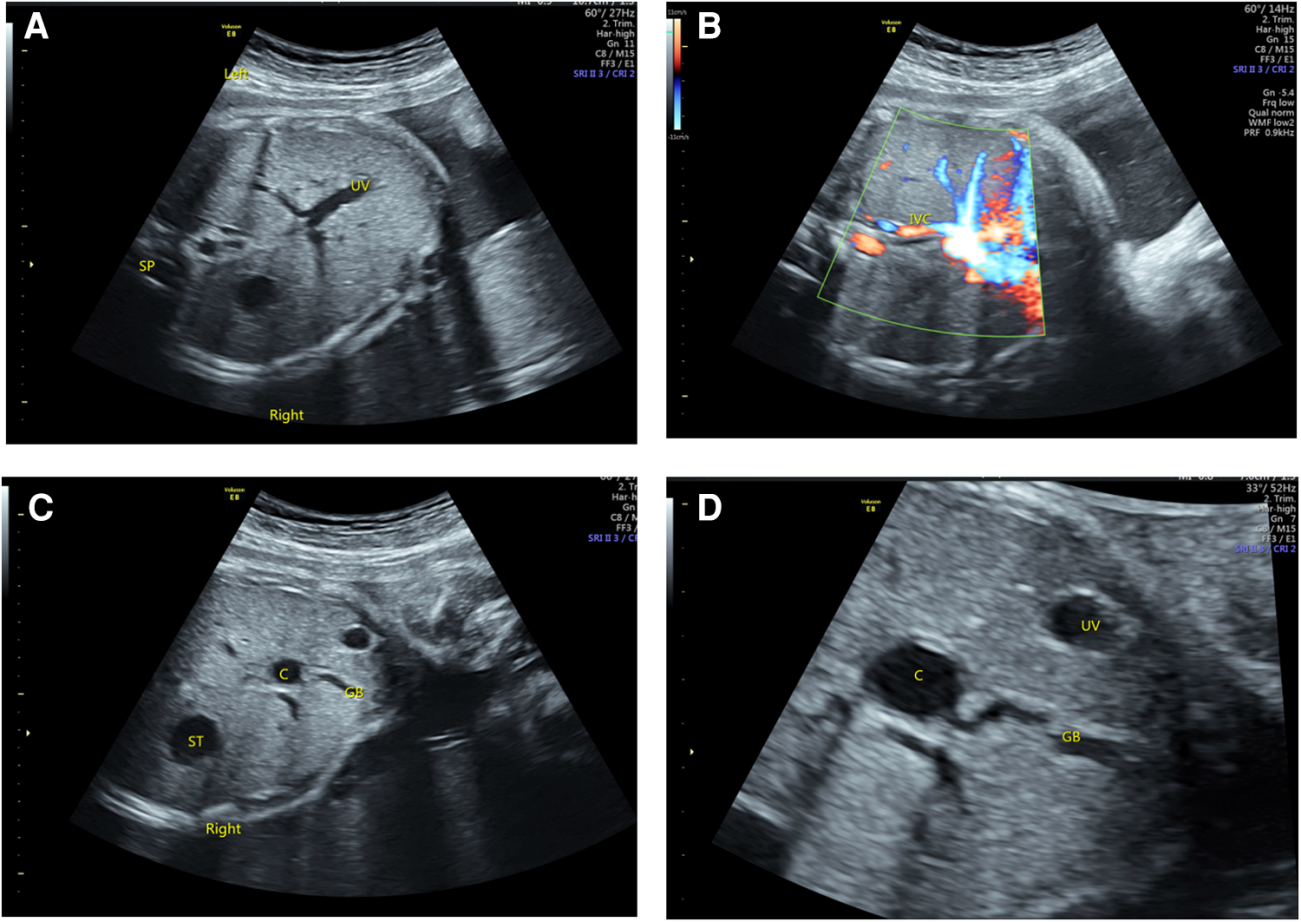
Ultrasonography of BASM in LAI fetuses. (A) The right-sided stomach and the portal vein branch show a “T” sign. (B) The IVC was small and flowed back to the right atrium after collecting hepatic vein blood. (C,D) A cystic structure was observed in the liver hilum, which was related to the gallbladder.
